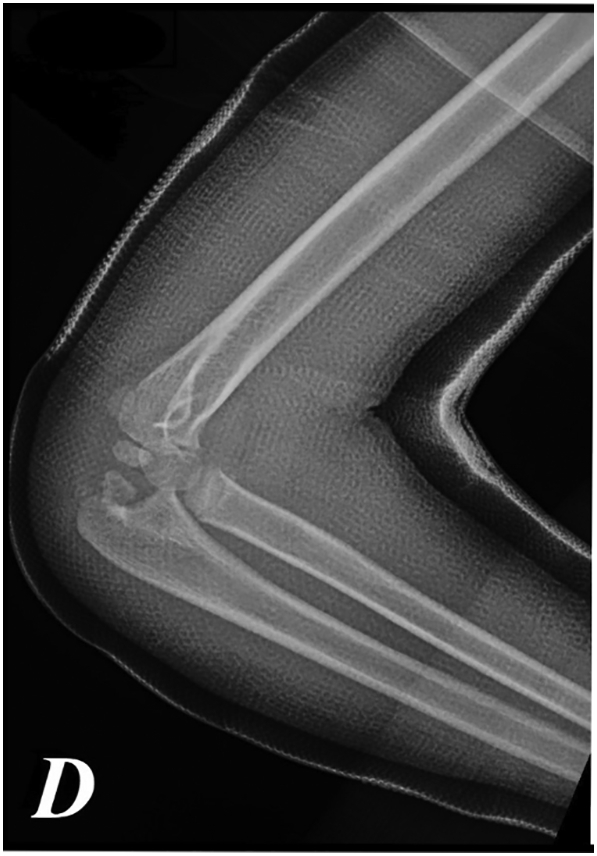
Elbow X-ray after a closed reduction attempt.
